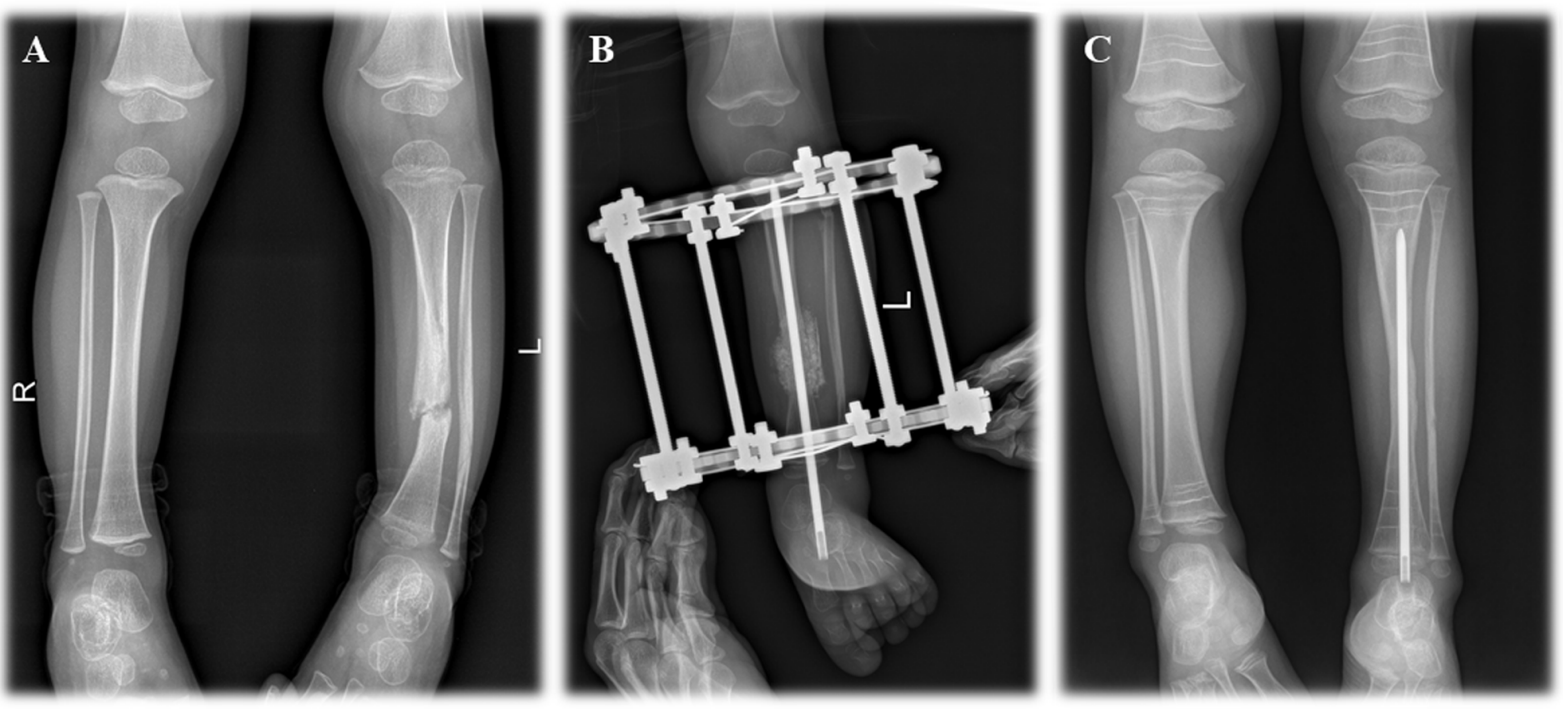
Preoperative and postoperative X-rays of combined surgery without cross-union. A preoperative X-ray; B postoperative X-ray within 7 days; C postoperative X-ray after primary union
